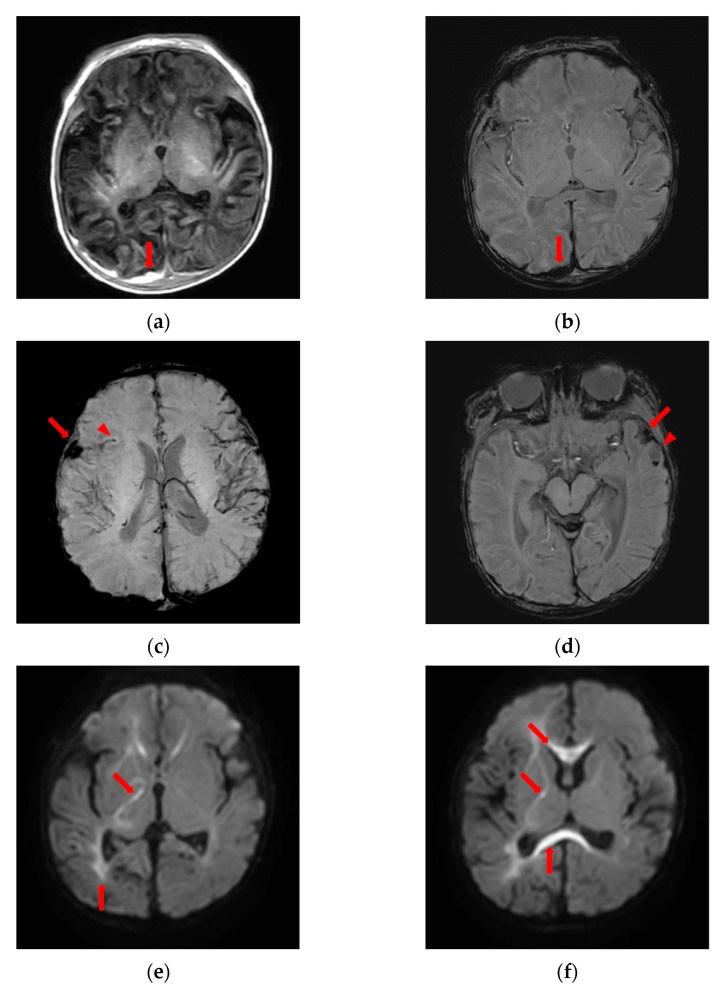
Brain magnetic resonance imaging suggesting abusive head trauma. (a) High signal intensity on T1-weighted image and (b) low signal intensity on susceptibility-weighted image, subdural fluid collection along the posterior aspect of the falx cerebri and occipital convexity implying subacute subdural hemorrhage (arrows); (c) low signal intensity in the right sylvian cistern (arrow), right sylvian fissure (arrowhead) and (d) left sylvian cistern (arrow) implying subarachnoid hemorrhage on susceptibility-weighted image; (d) low signal intensity with fluid-fluid level (arrowhead) along the anterior side of left temporal lobe implying acute hemorrhage on susceptibility-weighted image; (e,f) diffusion restriction in periventricular white matter, corpus callosum, right thalamus, and internal and external capsules on diffusion-weighted image (arrows).
